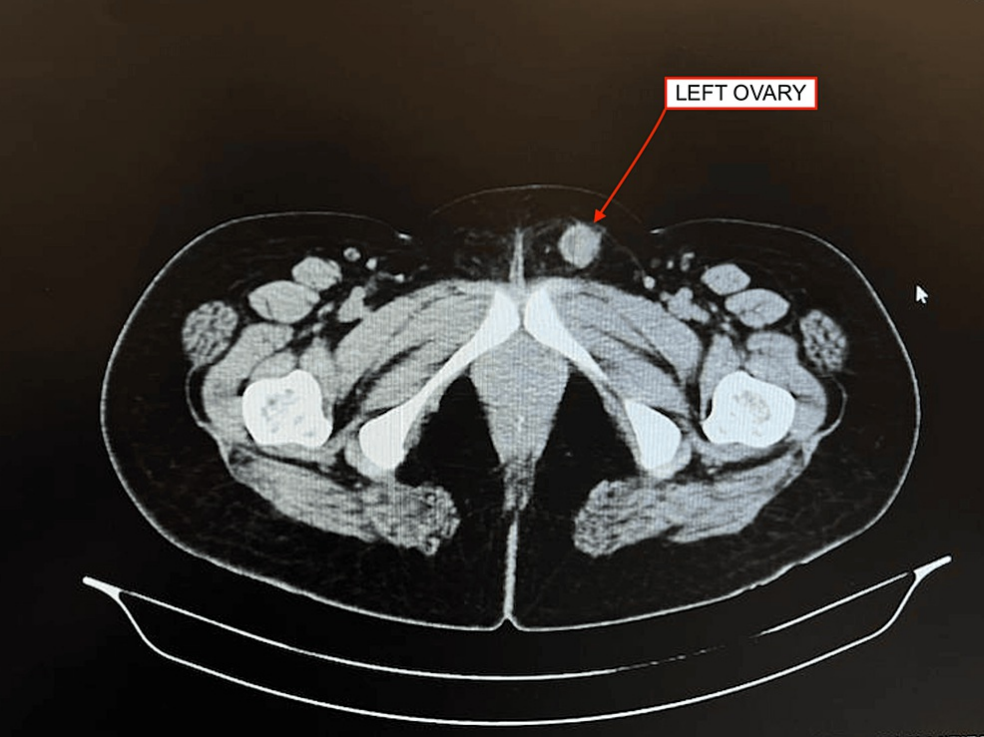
CECT of abdomen and pelvis confirms left-sided inguinal hernia with the left ovary and its mesovarium as content CECT: Contrast-enhanced computed tomography
